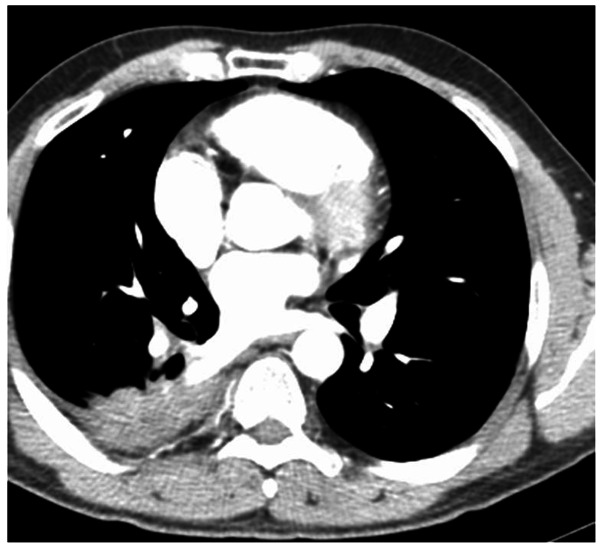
Total body computed tomography (CT) showing a lung mass located in the apical and postero-basal portion of the right lung.
